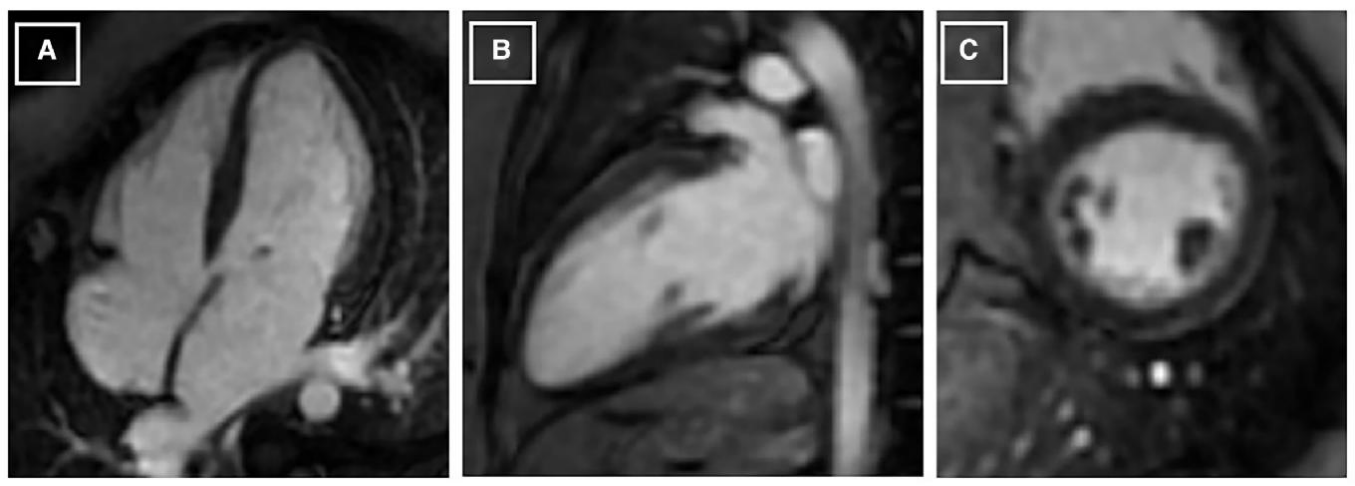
Cardiac magnetic resonance imaging early after contrast (gadolinium) showing no enhancement (Panel *A*, *B* and *C*).
